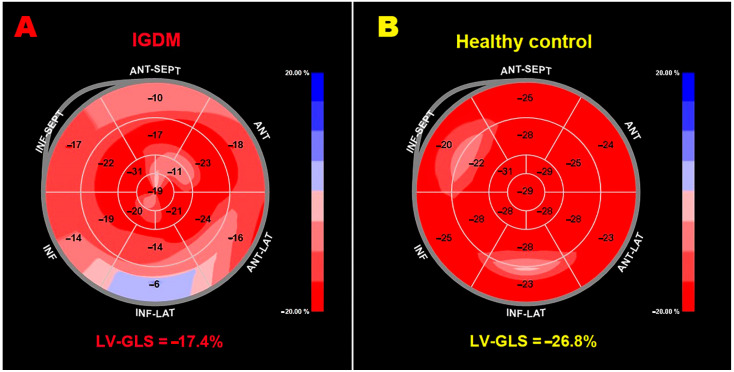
Representative examples of LV-GLS bull’s eye plot assessed by 2D-STE analysis in the perinatal period in an infant born to mother with GDM (A) and in an infant born to mother with uncomplicated pregnancy (B). Note—2D, two-dimensional; GDM, gestational diabetes mellitus; GLS, global longitudinal strain; IGDM, infant born to gestational diabetic mother; LV, left ventricular; STE, speckle tracking echocardiography.
